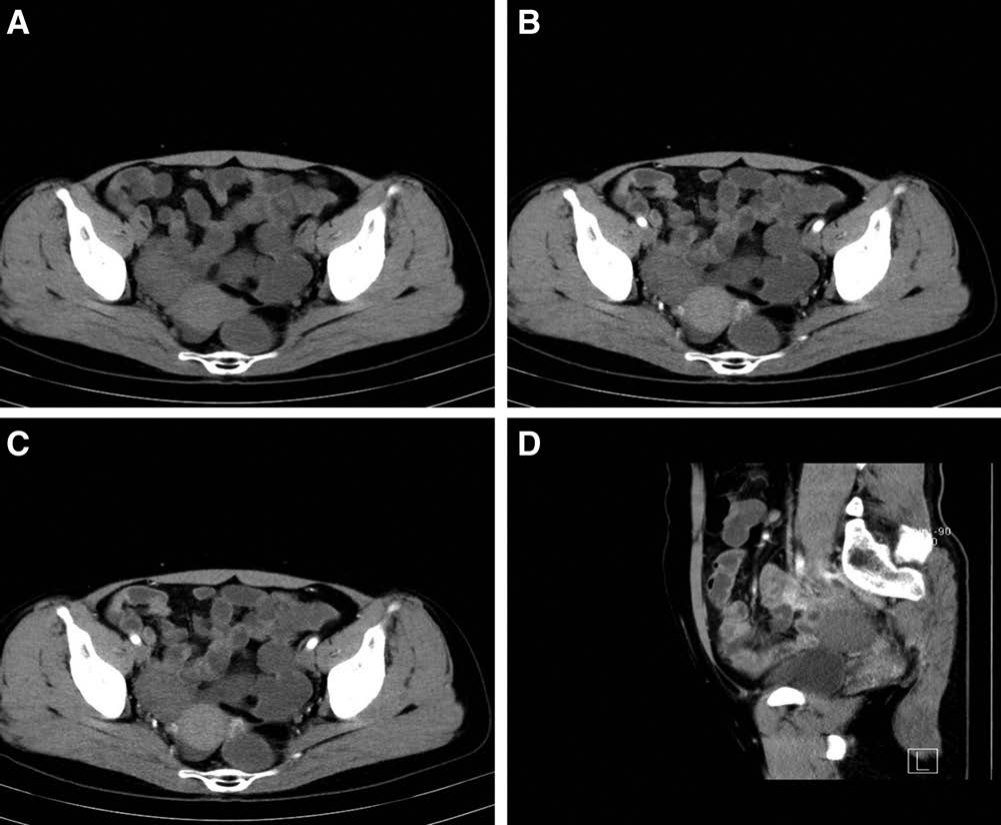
CT images of the mass. Plain CT scan showed a low density tumor with well-demarcated margin in the broad ligament (A). The neoplasm was mildly enhanced in both arterial phase (B) and venous phase (C). Three-dimensional reconstruction of the lesion on CT (D). CT = computed tomography.
